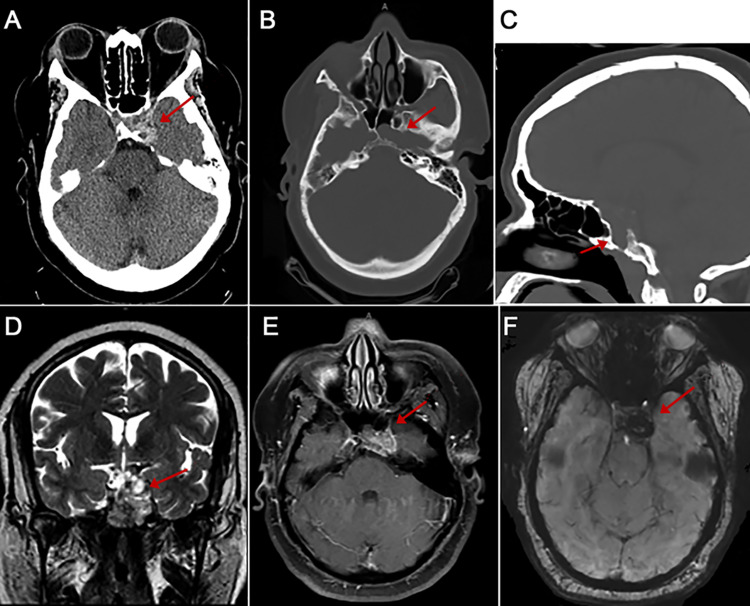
Imaging demonstrating hemorrhagic clival chordoma arising from the clivus. Computed tomography (CT) scans show the lesion (red arrows). (A, B) Axial images showing hyperdense lesion with extensive osseous erosion of the left sphenoid sinus and petrous bone. (C) Sagittal image demonstrating erosion of the sella and skull base. Magnetic resonance imaging demonstrating the lesion (red arrows). Axial coronal T2-weighted (D), axial T1-weighted with contrast (E), and axial gradient-recalled echo (F) magnetic resonance imaging demonstrating a heterogeneous enhancing hemorrhagic mass within the left cavernous sinus and sella and encasement of the cavernous internal carotid artery.
